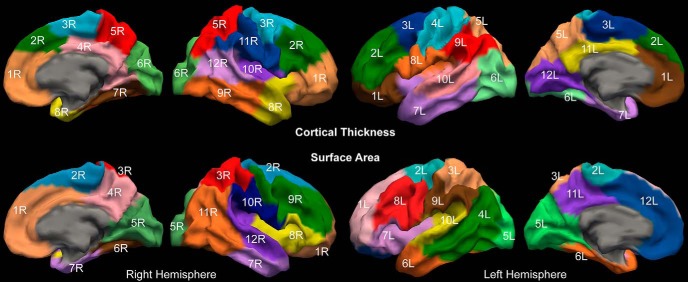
Summary of genetic parcellation of the CT and SA. Clusters are labeled 1L to 12L for the left hemisphere and 1R to 12R for the right one. Extended Data Figures 2-1, 2-2, 2-3, 2-4 describe the genetic clusters used in this analysis.
